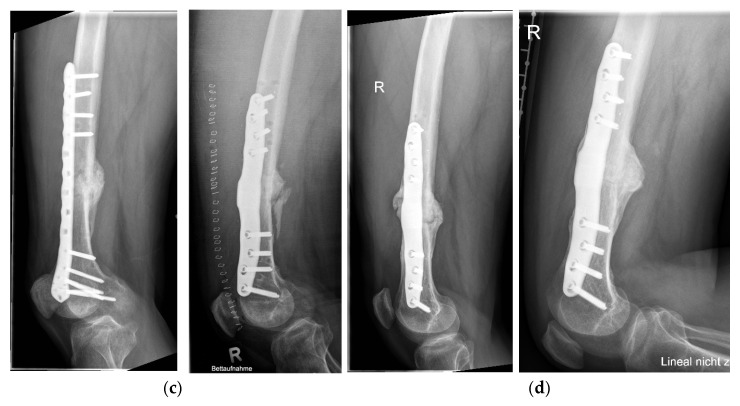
The figure shows the series of plain radiographs during very slow healing. Axial and anteroposterior radiographs showing the pre- (a) and postoperative situation (b), the situation at 3 years with few radiographic healing signs (c) and the late result after 10.5 years (d).
